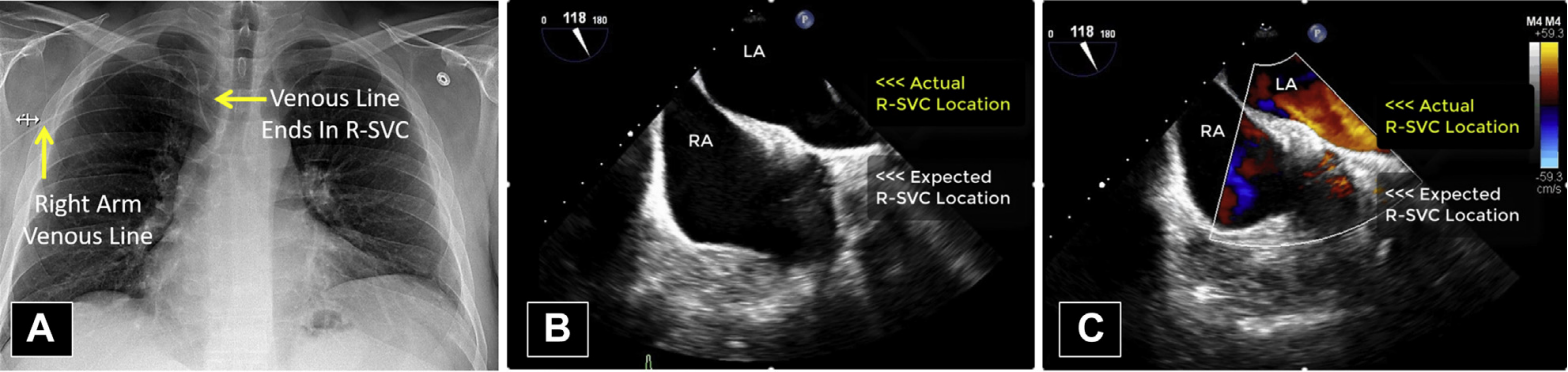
Case 5: R-SVC draining into the LA. Chest x-ray with anteroposterior projection (A) and TEE with midesophageal view at 118° in grayscale (B) and with color Doppler (C). A chest x-ray (A) taken to confirm the position of a right arm venous line confirms the presence of an R-SVC. However, TEE (B, C) reveals that the R-SVC in this patient is not in the usual location; instead, it is located further posterior where it drains into the LA. Videos 9 and 10 correspond to this figure.
